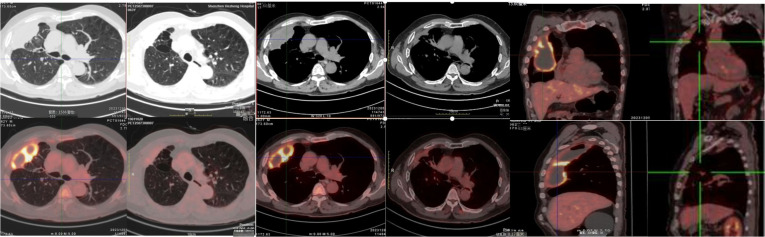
Comparison of PET/CT findings obtained after completion of chemoimmunotherapy (December 2023) and at the latest follow-up after approximately 2 years of maintenance adebrelimab therapy (July 2025). For each paired image, the left panel corresponds to December 2023 and the right panel corresponds to July 2025. PET/CT at the latest follow-up demonstrated sustained disease control with no evidence of disease progression.
